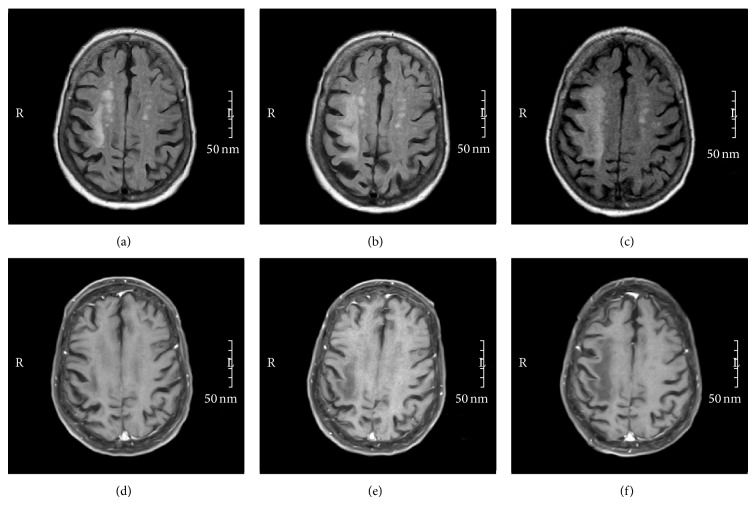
Top row: MRI head, axial T2-FLAIR. Bottom row: MRI head, T1-post gadolinium contrast in same chronological order (right to left). Note the prominent progressive asymmetric subcortical T2-FLAIR signal abnormality over the course of 12 weeks with noted lack of pathological enhancement during the same time window. (Left) image: week 1, middle: week 6, and right: week 12. Lumbar puncture was obtained after the second MRI (middle).
